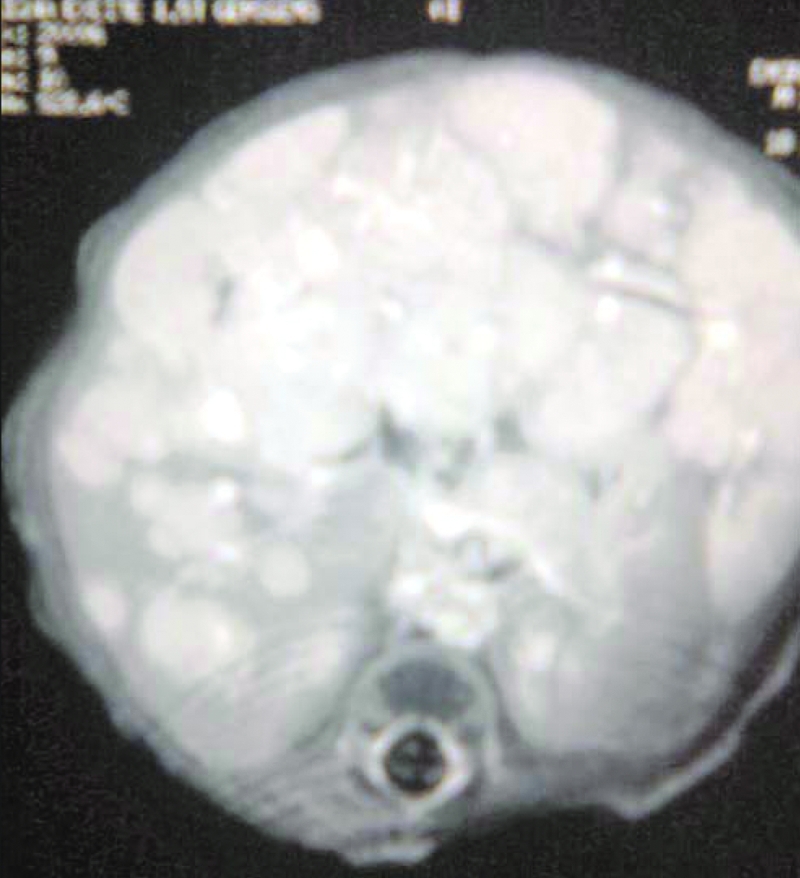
Abdominal MRI showing multiple hepatic nodules
